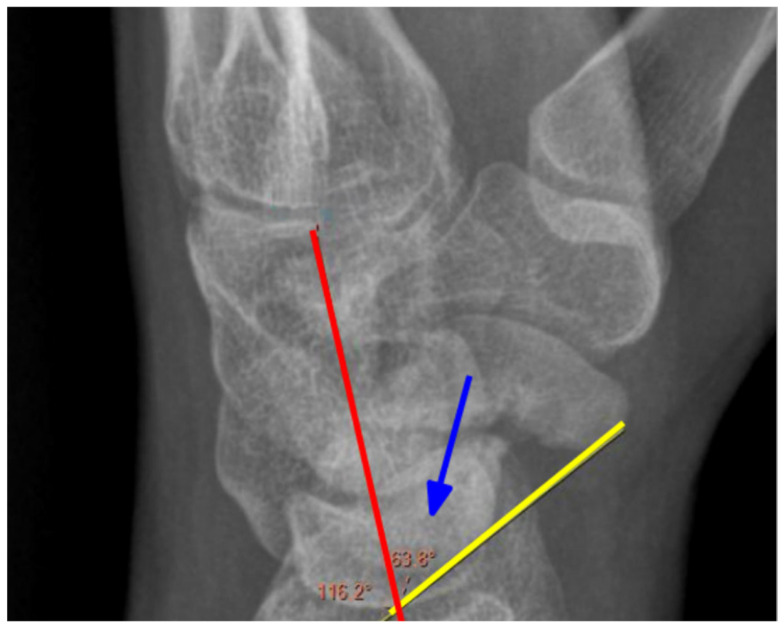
Measurement of the SLA angle. Blue arrow—angle, yellow line—the axis of the scaphoid, red line—the axis of the lunate. X-ray of the right hand, lateral view.
